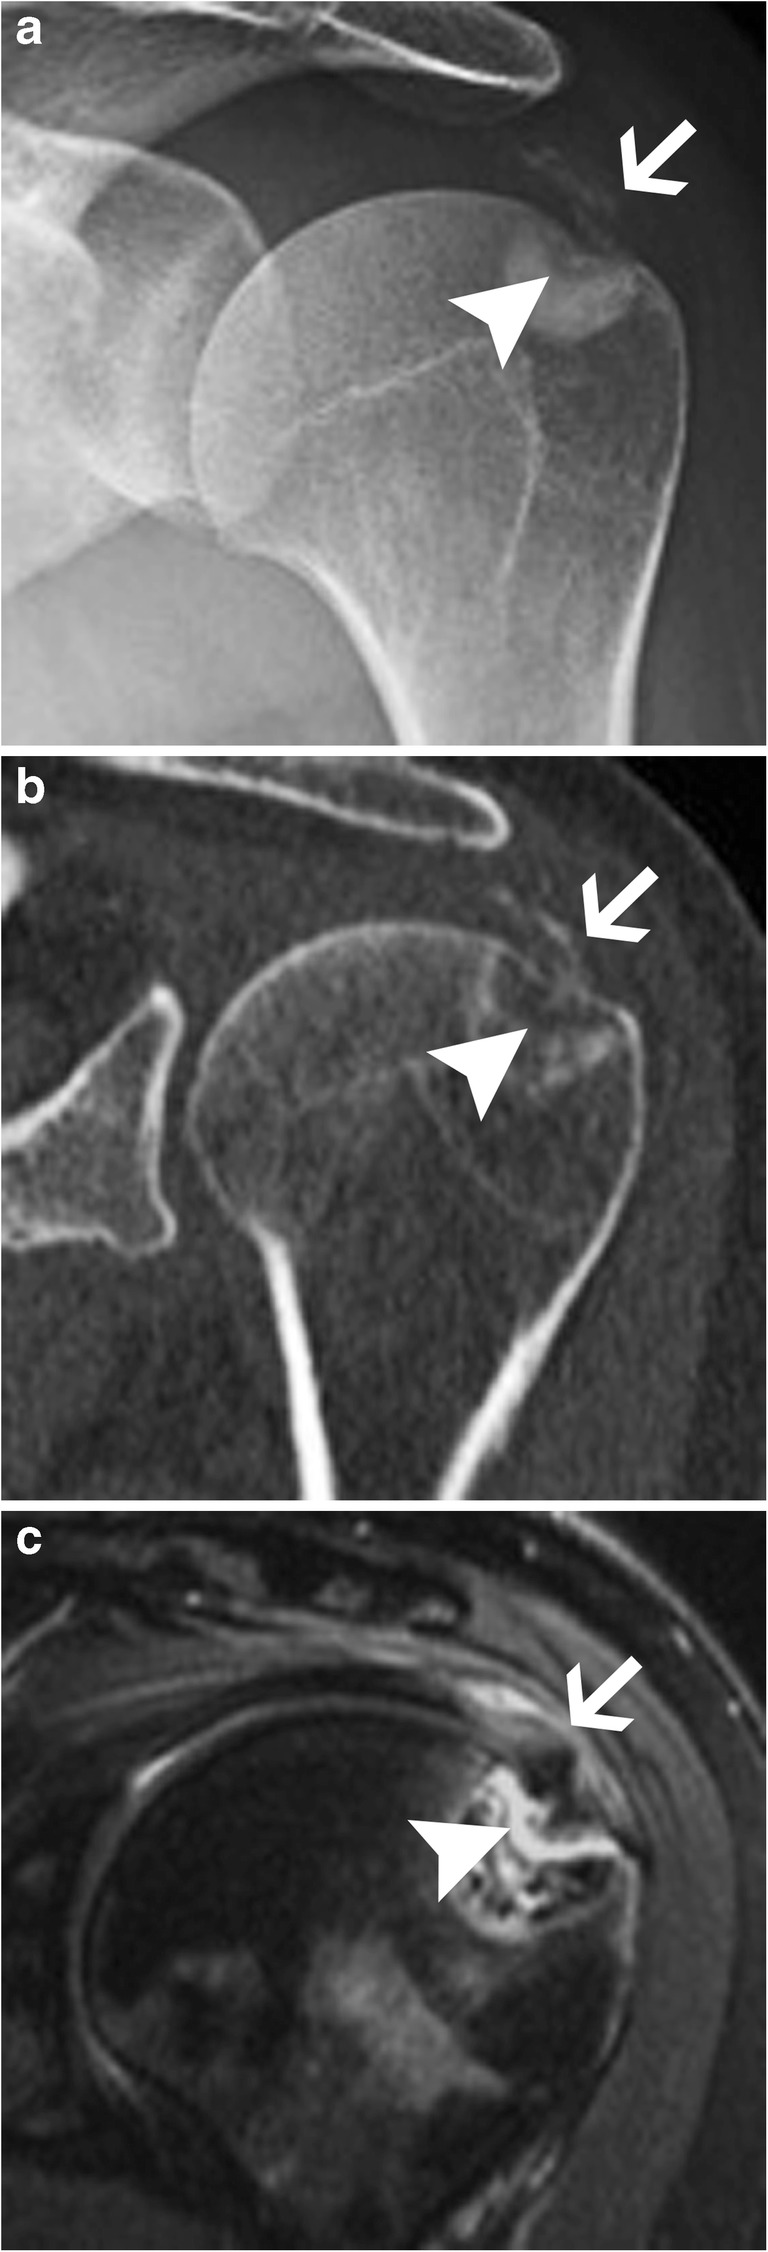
Supraspinatus calcifying tendinopathy with intraosseous extension. (a) Frontal radiograph, (b) coronal reformat CT image and (c) coronal fat-saturated T2-weighted image show supraspinatus amorphous calcifications (arrows) with intraosseous extension causing erosions (arrowheads). Note the sclerosis around the erosion, hyperdense in (a) and (b) and hypointense in (c)
